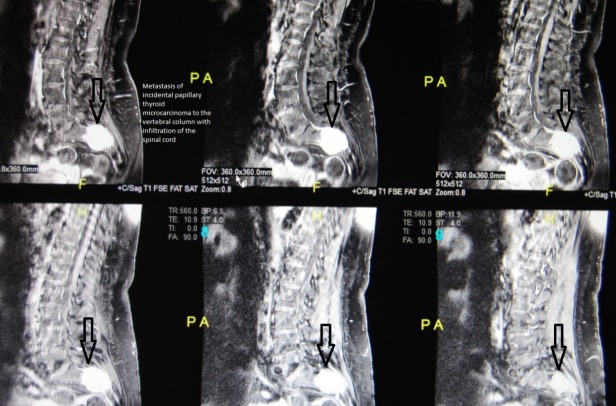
MRI sagittal scan showing area of high signal (arrows) corresponding to spinal metastasis of ITC in a 61-year-old female.
